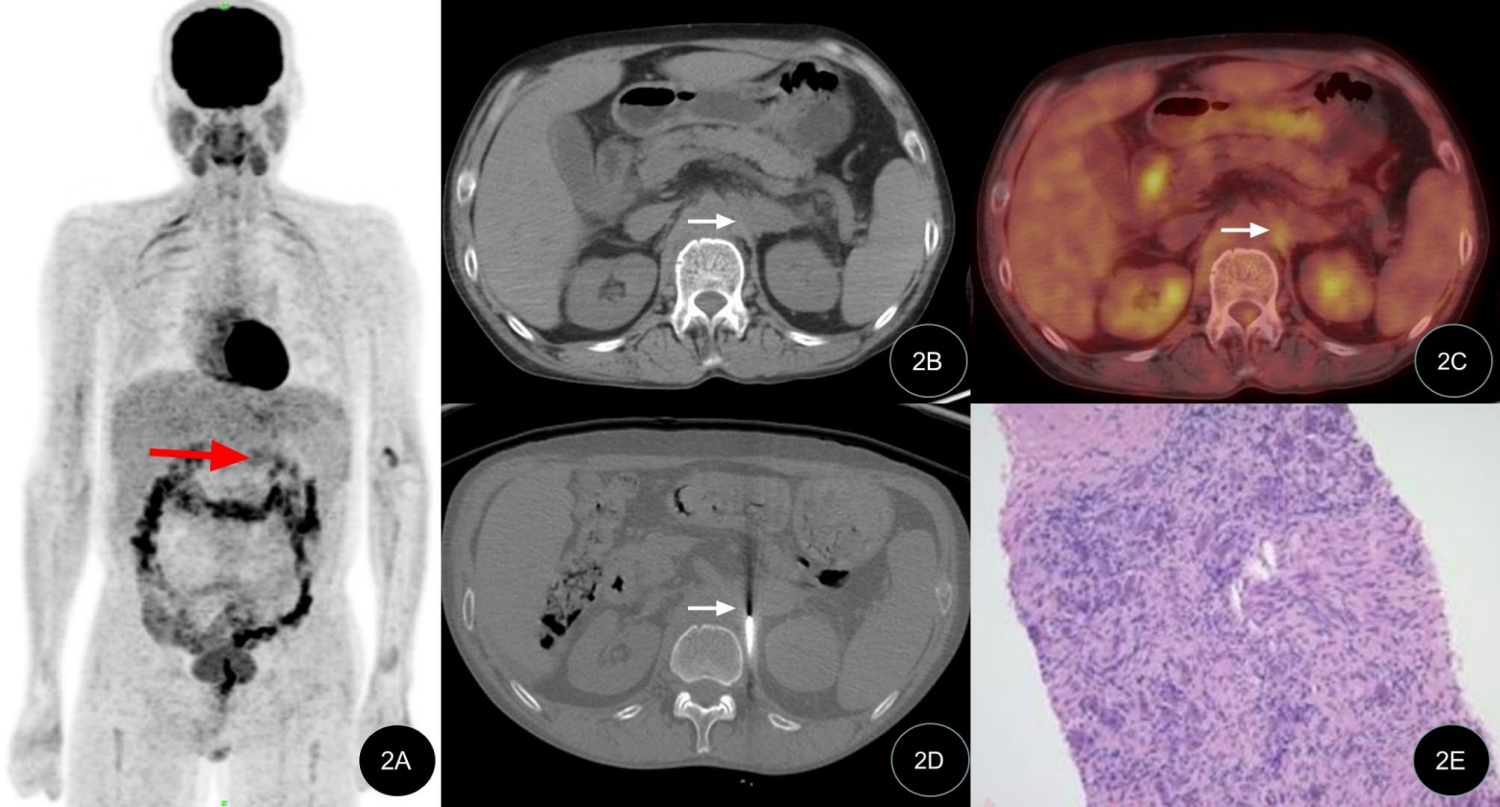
PET/CT-guided targeted retroperitoneal masses biopsy The patient (49-year-old male) was admitted due to abdominaldistension. The PET/CT MIP image, PET/CT fusion CT image, puncture biopsy image, and biopsy pathological image are shown. 2A MIP image shows abnormal metabolism increase at the left side of the lumbar spine (indicated by red arrow); 2B, 2C PET/CT fusion CT image shows increased metabolism at the retroperitoneal mass; 2D Puncture biopsy of the target area of the retroperitoneal mass under PET/CT guidance; 2E Pathological examination shows proliferative fibrotic tissue (HE × 100)
